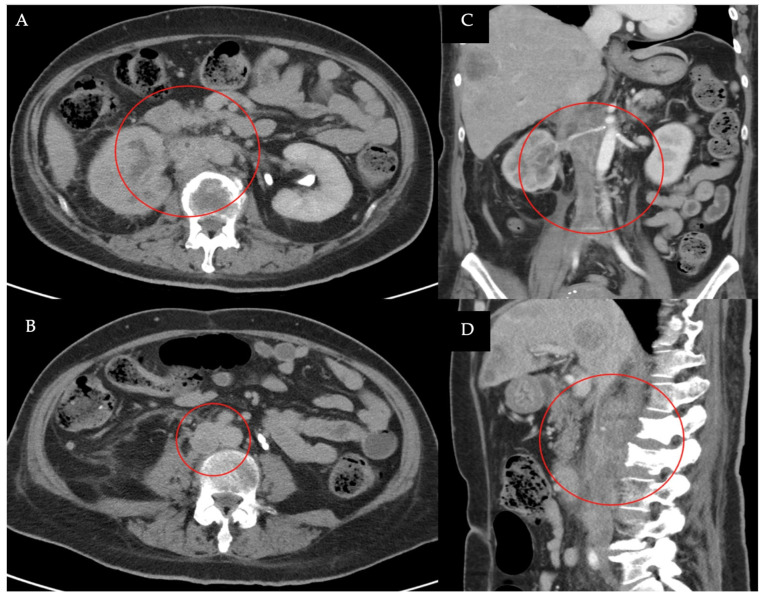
CT examination with the contrast enhanced: (A) Axial CT image showed heterogenous contrast-enhancing mass from right renal invaded the IVC (red circle); (B,D) Coronal CT and sagittal CT images showed heterogenous contrast-enhancing mass was noted in the IVC from CIVs to the level of renal veins (red circle); (C) Axial CT image showed irregular mass obstruction was noted in IVC at the level of bifurcation of CIVs (red circle).
